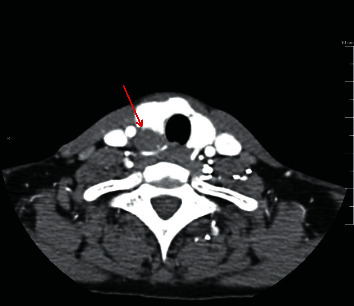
Axial CT-neck with contrast reveals an enlarged right inferior parathyroid gland (*red arrow*).
